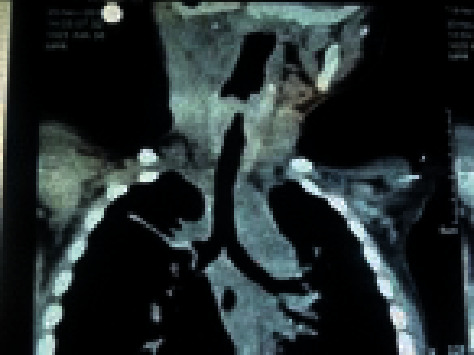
Postoperarative appearance on coronal CT images, after surgery for acquired subglottic stenosis; note the adequacy of the airway.
